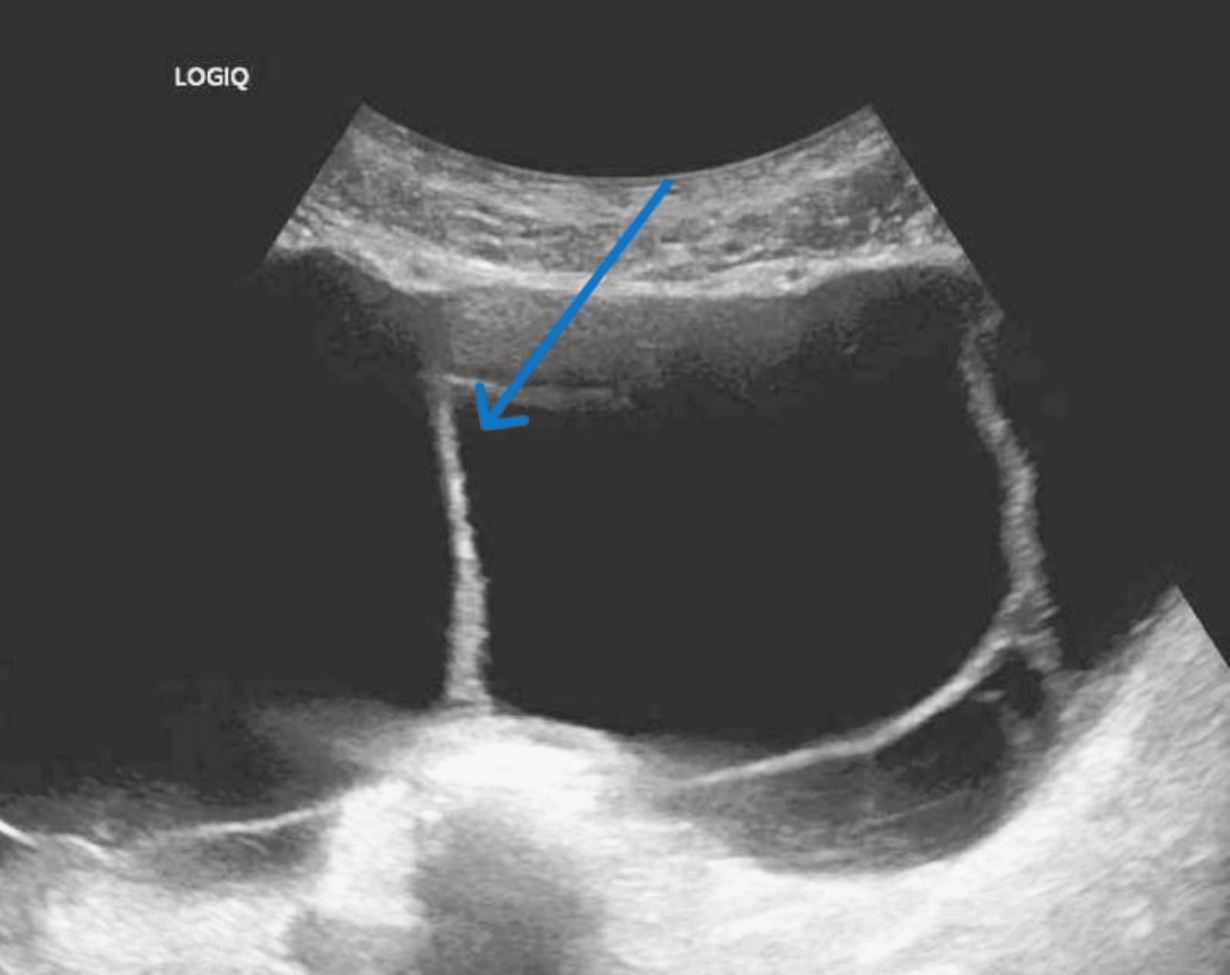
Suprapubic ultrasound demonstrating the patient’s loculations prior to drain placement and tPA dwells. Pelvic portion of his right-sided collection. tPA: alteplase
